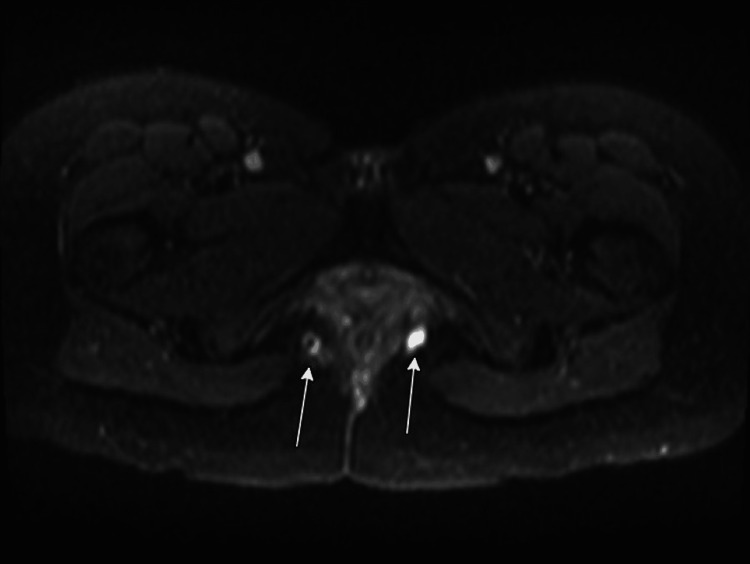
MRI demonstrating complex fistula (yellow) with bilateral (L > R), evolving ischiorectal abscesses (white). During the patient’s first procedure at our institution, surgeons located the internal opening of the abscess in the posterior midline, just distal to the dentate line. It was found to have extension from the posterior midline to both the left and right.
